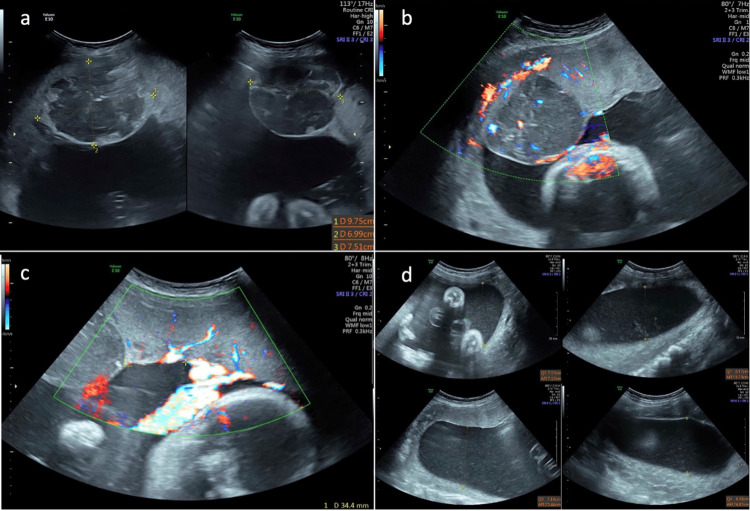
Ultrasound image at 26 weeks gestation showing a heterogeneous placental mass (a), tumor’s feeding vessels (b), distance from placental cord insert (c), and polyhydramnios (d).
